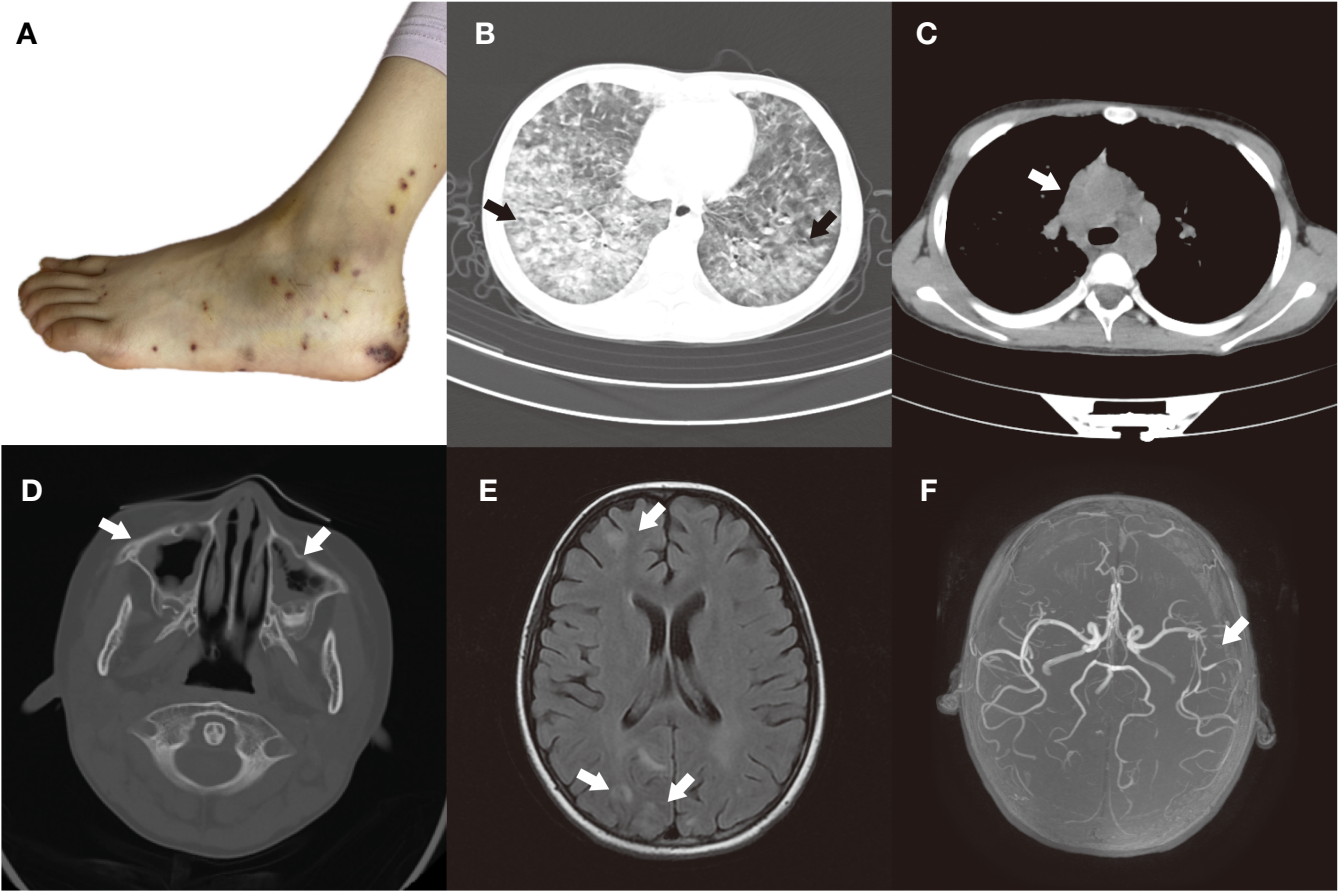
(A) Foot rash, partially ulcerated and scabbed. (B, C) Before treatment, the HRCT scan of the chest showed multiple patchy and nodular lesions with unclear boundaries, indicating high-density shadows in both lungs. And multiple enlarged lymph nodes were observed in the mediastinum and bilateral hilar regions. (D) Before treatment, the CT plain scan of the paranasal sinuses revealed a notable thickening of the mucosa in the bilateral maxillary, ethmoid, sphenoid, and frontal sinuses. (E) Before treatment, the MRI scan of the brain showed multiple abnormal signal shadows in the bilateral cerebral cortex. (F) Before treatment, the MRA revealed moderate to severe stenosis in the middle cerebral artery segment M2 of the left middle cerebral artery.
